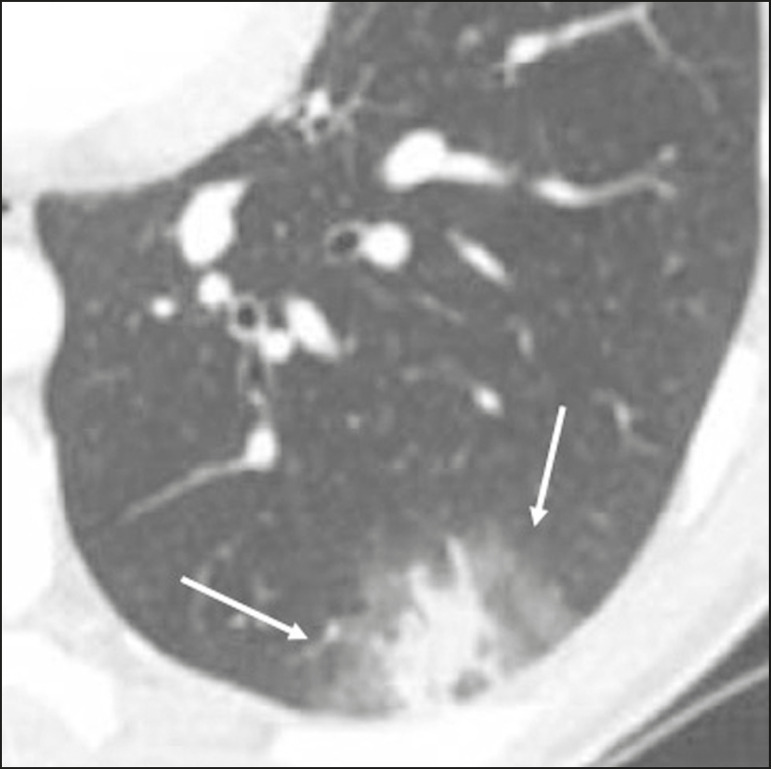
Chest CT scan showing a halo sign (arrows), characterized by pulmonary nodular consolidation with ground-glass halo (arrows), in a patient with COVID-19.
